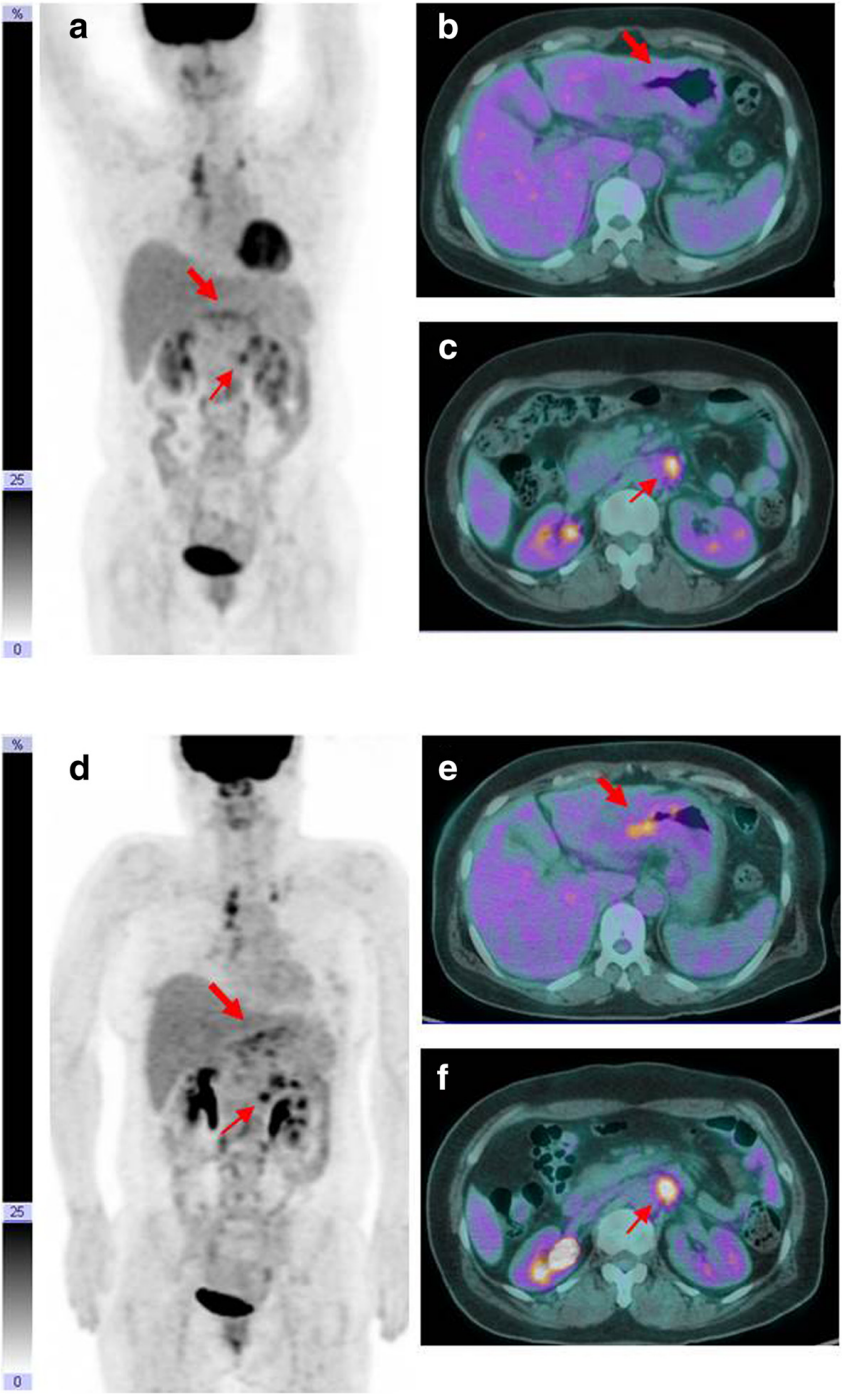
A 52-year-old woman with gastric cancer and multiple lymph nodes metastases. a, b and c Before administration of pioglitazone, SUV_mean_ and isocontour of gastric cancer in ^18^F-FDG PET/CT image were 3.09 and 6824.52 Bq/mL. d, e and f After administration of pioglitazone, they were increased to 3.78 and 9480.92 Bq/mL. In both maximum-intensity-projection and axial PET/CT images, glucose metabolism in gastric cancer and metastatic paraaortic lymph node shows increasing pattern on the next day after the use of pioglitazone. This patient also has both supraclavicular and right mediastinal lymph nodes metastases
